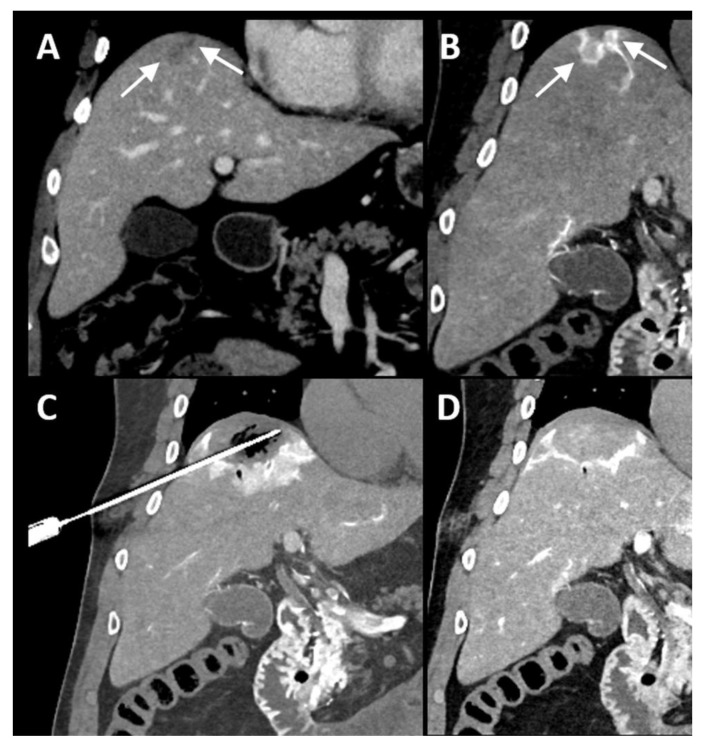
Identification of colorectal liver metastasis. Two adjacent colorectal liver metastases (arrows) in the hepatic dome, hardly visible on diagnostic CT-imaging in portal venous phase (A). After catheterization of the common hepatic artery, the lesions (arrows) became clearly visible as enhancing rings after injection of 20 mL of contrast agent (Visipaque™, iodixanol, 320 mg Iodine/mL, GE Healthcare Inc., Marlborough, MA, USA) just prior to probe placement (B). At the early end of microwave delivery (65 W, 7 min), 15 mL of contrast agent was injected, confirming adequate ablation coverage of both tumors (C), and another 20 mL was injected after complete ablation to assess the ablation zone and potential complications (D).
